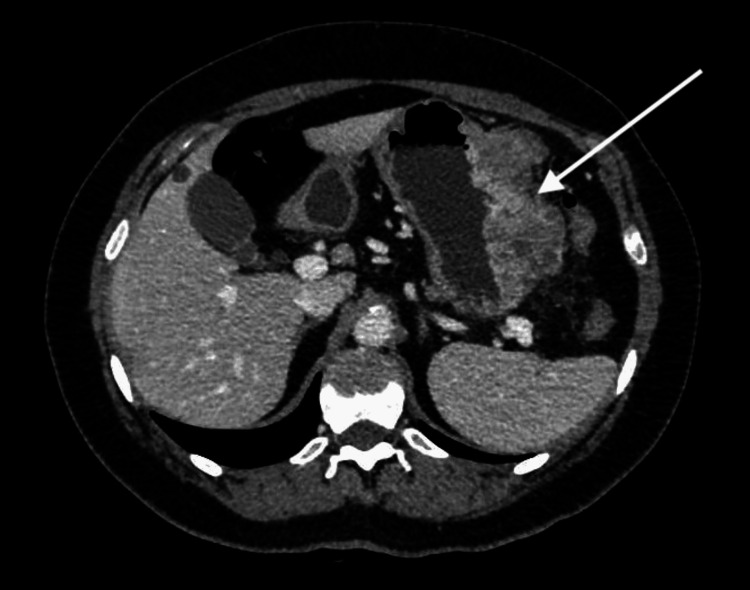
Preoperative abdominal CT scan (venous phase, axial plane): a large mass arises from the greater curve of the stomach (white arrow) CT: Computed Tomography
